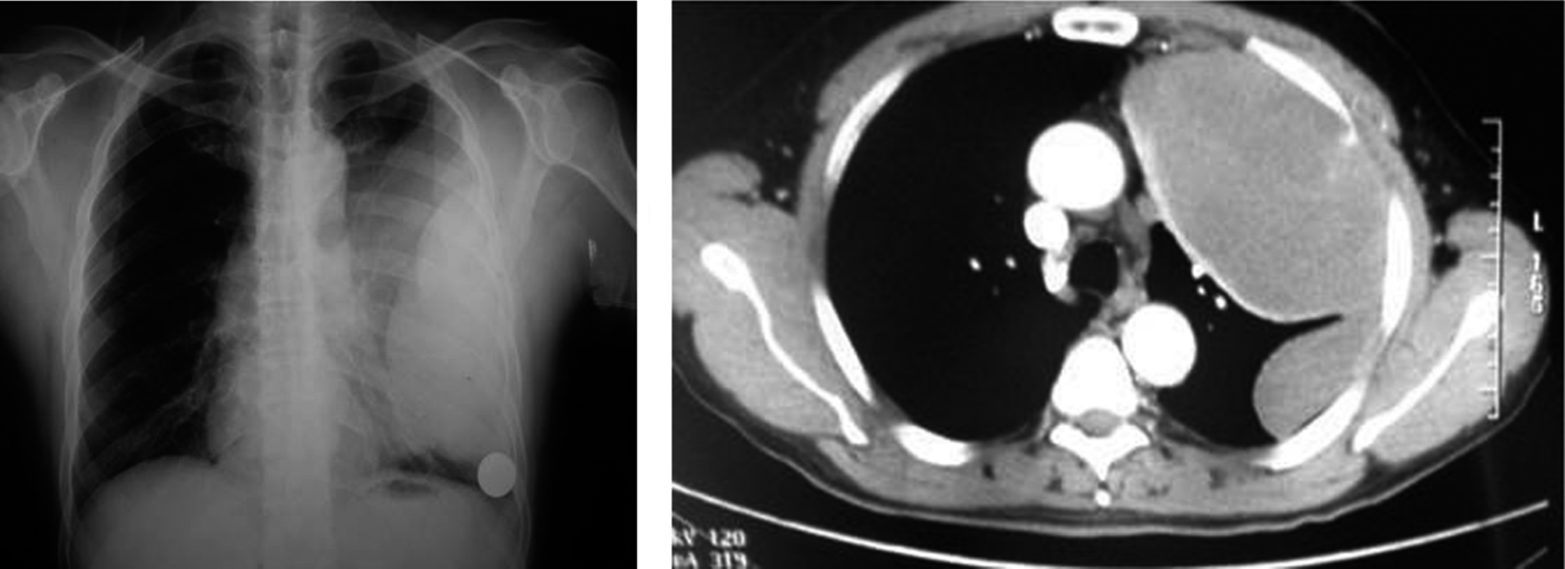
(A) Chest radiograph and (B) computed tomography at the time of presentation in 2015 showing a pleural‐based opacity involving middle and lower zones of left hemithorax.
